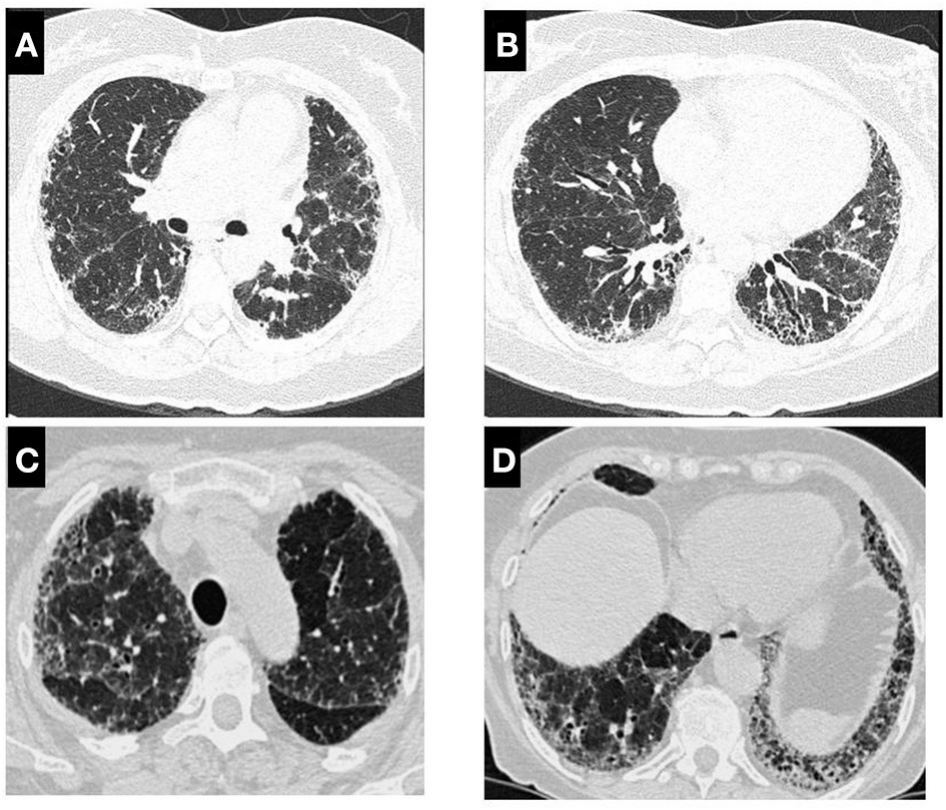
High-resolution computed tomography scan in patients with fibrotic HP. In (A), it is observed bilateral subpleural reticulation and in (B) traction bronchiectasis, honey combing and discrete ground-glass opacification and volume loss in left lung; (C) show lung fibrosis and areas of low attenuation and the same patient in (D) bronchiectasis and persistence of mosaic attenuation.
